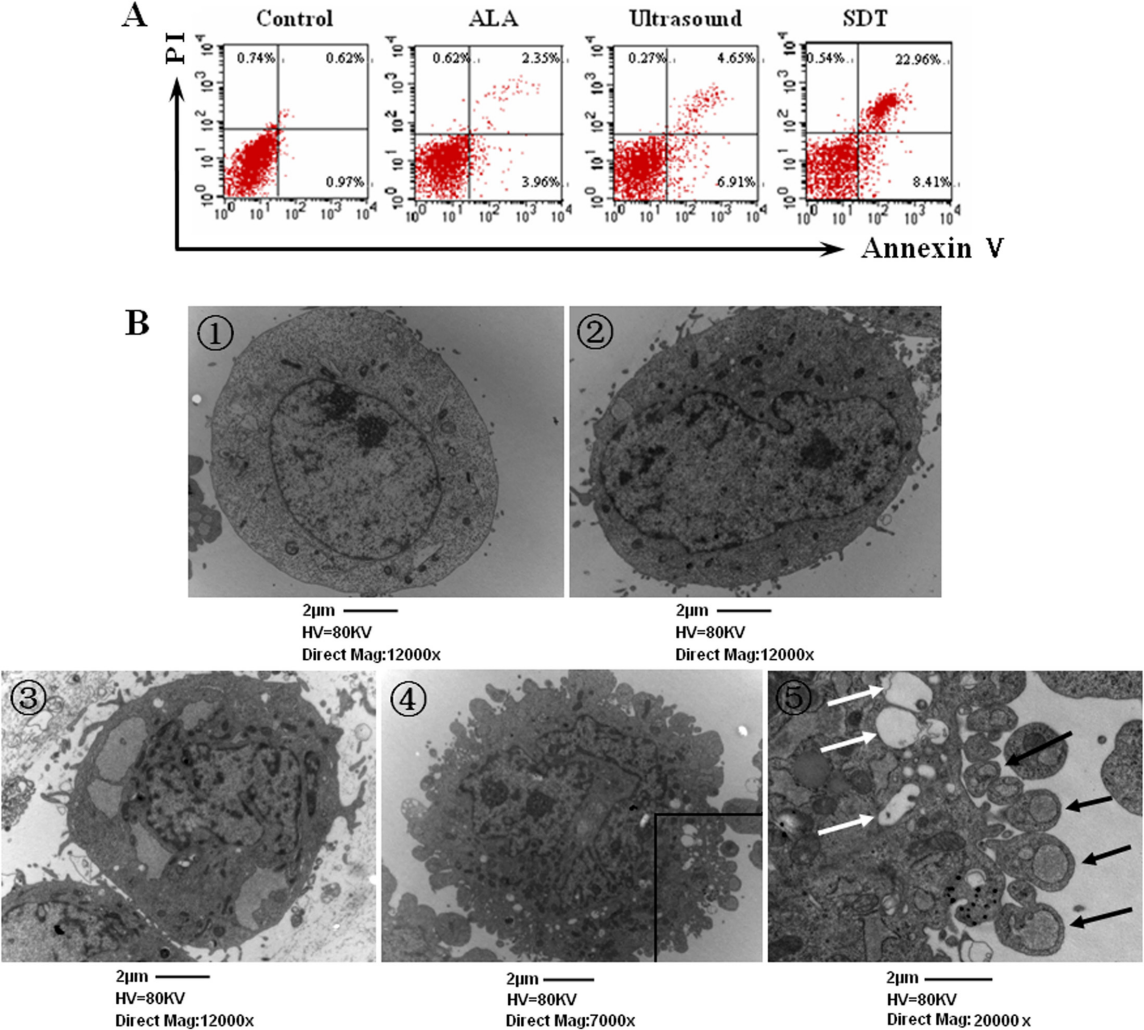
ALA-SDT induces osteosarcoma cell apotosis in vitro. UMR-106 cells were treated with vehicle (Control), 2 mM 5-ALA or/and ultrasound for 7 minutes, cultured for 6 hours and the percentages of apoptotic cells were determined by flow cytometry. In addition, the cells damages of individual groups of cells were characterized by TEM. Data are representative images of individual groups of cells from three separate experiments. A. Flow cytometry analysis of apoptotic cells. B. TEM analysis of cell damages (Magnification×20000). The white arrows indicate apoptotic bodies.
